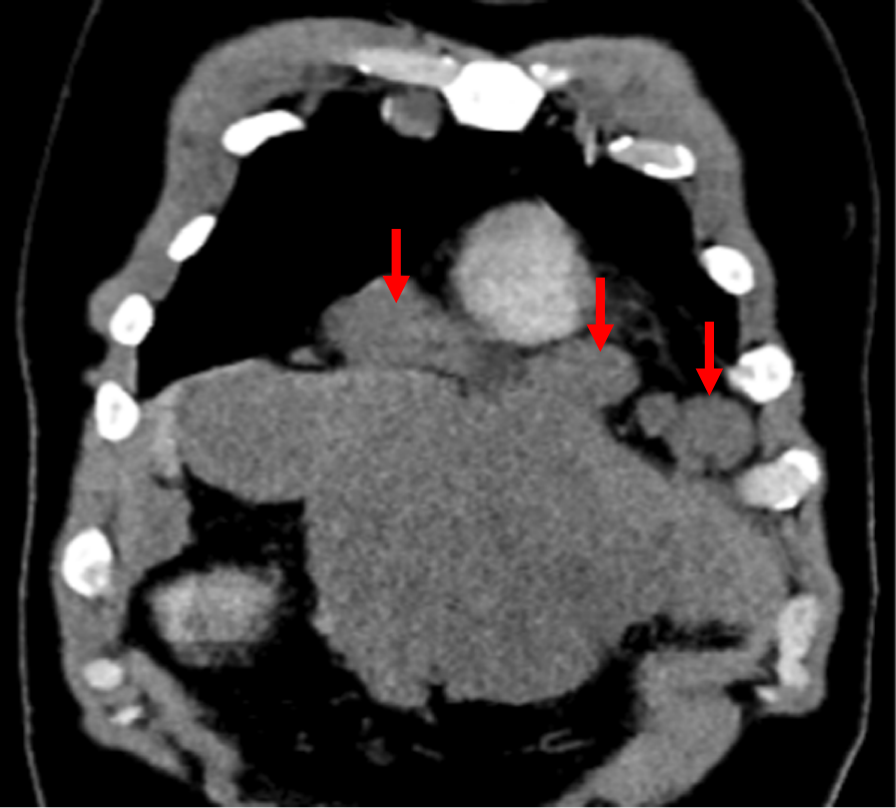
Case 3: coronal section of thoracoabdominal CT scan, showing multiple bilateral mediastinal adenopathies of anterior cardiophrenic angles (red arrows)
